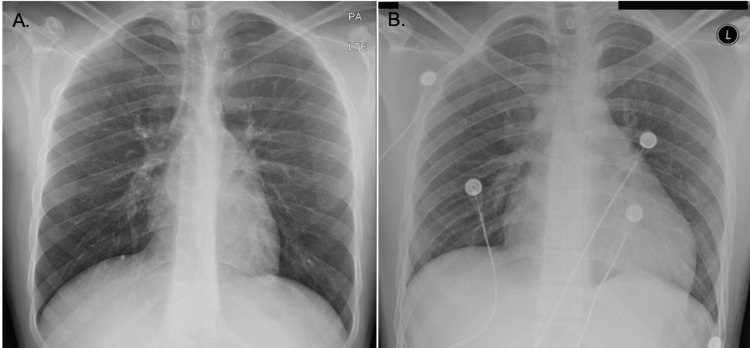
(A) Chest x-ray on the day of presentation, normal. (B) Chest x-ray on day 5 of admission showing enlargement of the cardiac silhouette.
